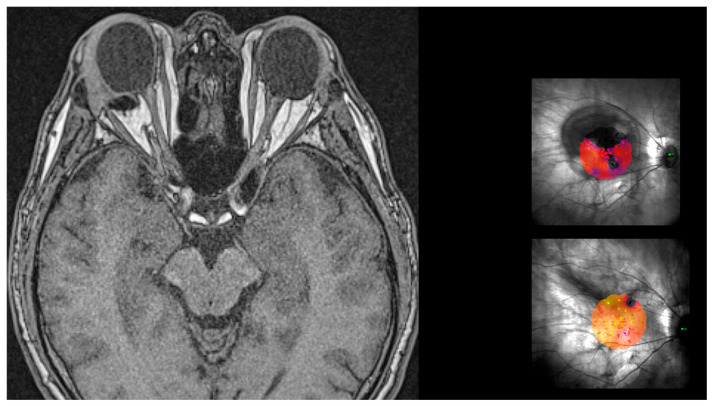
MRI of brain and orbits showing the shape of the head of the macular buckle behind the right eye. The buckle does not touch the optic nerve. This patient was operated on 11 years ago. The microperimetry on top shows the large scotoma prior to surgery and the one on the bottom shows the complete resolution of the scotoma after surgery.
